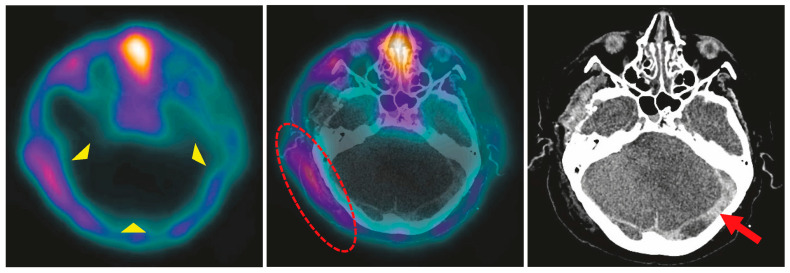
SPECT (left), SPECT/CT fusion (middle), and CT images (right) of patient no. 18. The axial SPECT reconstruction confirms the loss of perfusion in the cerebellum (yellow arrowheads). The tracer activity seen on the lateral planar image of this patient corresponds to hyperperfused, extracranial swollen tissue (red oval). Subarachnoid hemorrhage is present (red arrow).
